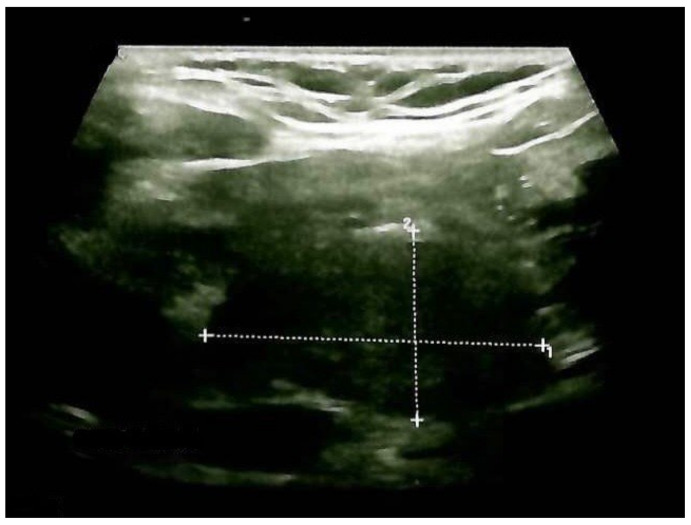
Anterior neck ultrasound: hypoechoic mass (ETTM) extending from the right thyroid lobe, with a structure similar to the thyroid and pre-tracheal extension of 3.66 by 2 by 2.6 cm (longitudinal plane).
